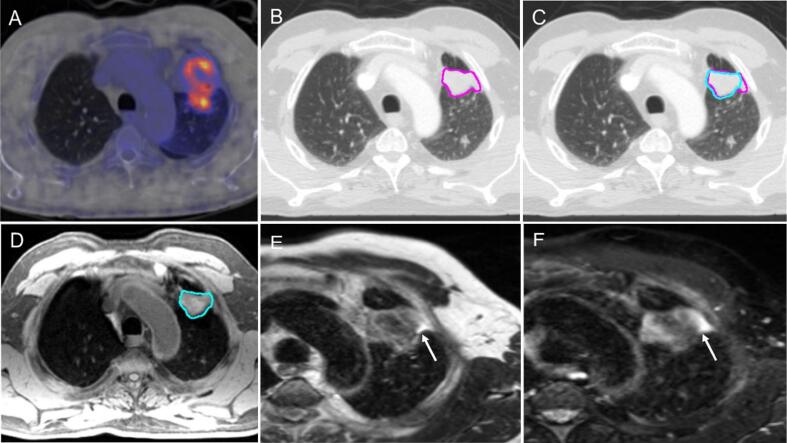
Tumour abutting the chest wall; (A) PET-CT scan, (B) CT in lung windows (L: −474 HU, W: 1500 HU) with CT consensus contour (pink), (C) CT scan in lung windows, with CT consensus contours (pink) and MR consensus contours (light blue), demonstrating more generous CT consensus contour at the chest wall/tumour interface; (D) fat-suppressed T_1_w Radial GRE with MR consensus contour (light blue), (E) T_2_w TSE non-fat-sat, (F) T_2_w TSE fat-sat. Arrows demonstrate difference in signal intensity between tumour and parenchymal/ pleural changes. (For interpretation of the references to colour in this figure legend, the reader is referred to the web version of this article.)
